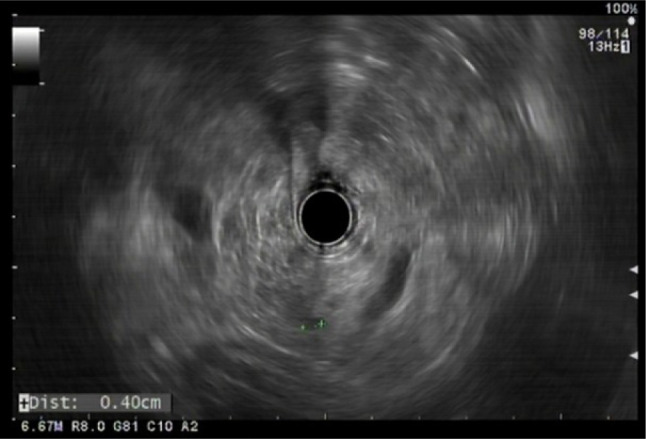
Endoscopic ultrasound of the heterozygous brother showing a 4 mm
